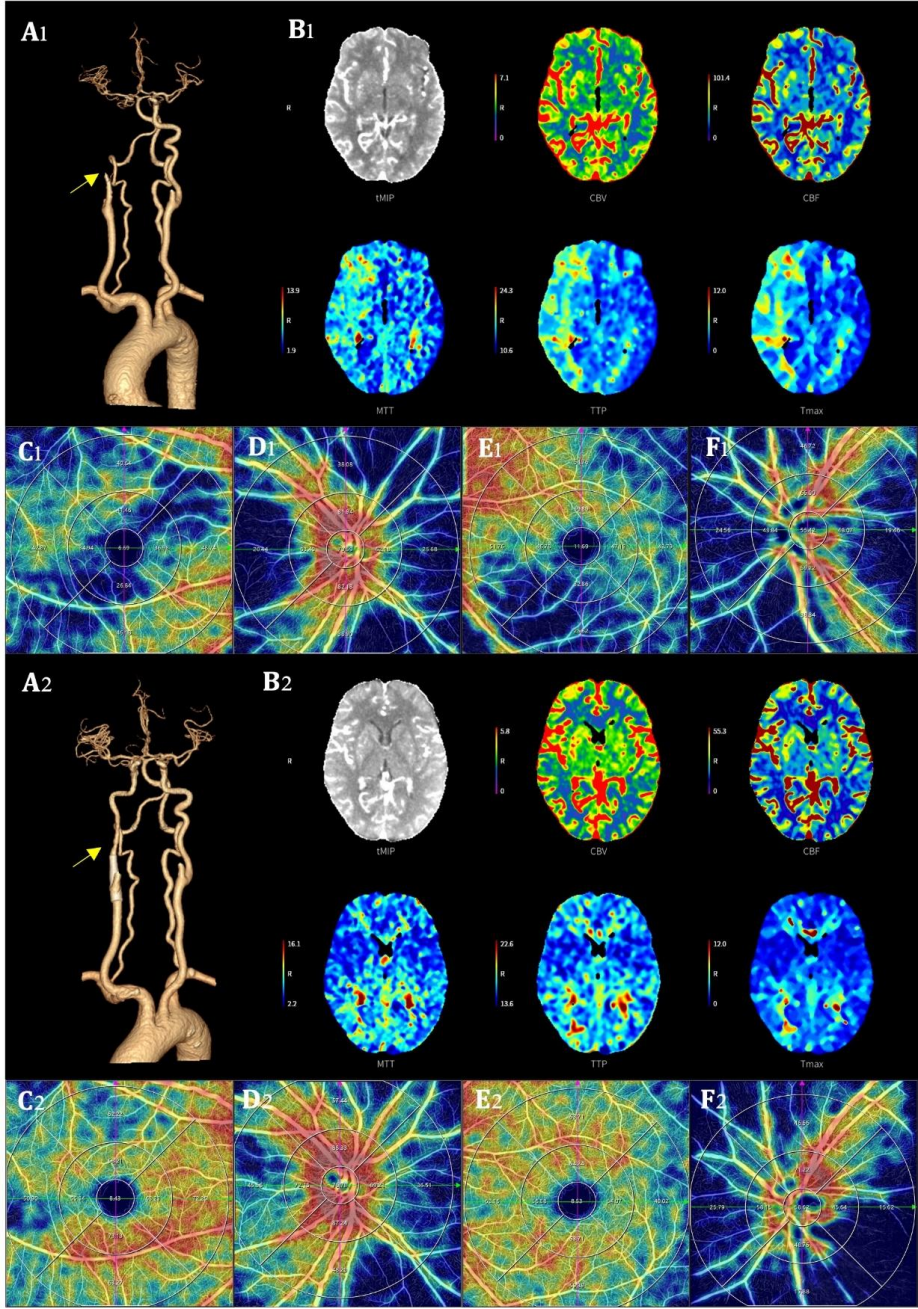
An example of OCTA findings in a CAS patient. A 66-year-old male accepted carotid artery stent surgery due to severe stenosis of the right carotid artery (>90%). Baseline (December 27^th^, 2023) CTA and CTP showed an occlusion of the right ICA (C2-C7 segments) (yellow arrow, A1), and extensive hypoperfusion in the right hemisphere (B1). In his pre-operative OCTA images, the SRCP VD in the macular (C1, E1) and optic disc (D1, F1) regions of both eyes were significantly reduced, especially seen in the right eye (C1*vs*. D1, E1*vs*. F1). Three days after the surgery (January 12^th^, 2024), the follow-up CTA image revealed that the occluded right ICA was successfully recanalized (yellow arrow, A2 *vs*. A1), and the baseline intracranial hypoperfusion was reversed to normal (B2 *vs*. B1). The post-operative OCTA images showed significant improvement of the SRCP VD of the macular (C2 *vs.* C1, E2 *vs.* E1) and optic disc areas (D2 *vs.* D1, F2 *vs.* F1) in his both eyes, indicating that the blood flow perfusion of the retina in both eyes has been restored. CAS, carotid artery stenosis; CTA, computer tomography angiography; CTP, computer tomography perfusion; ICA, internal carotid artery; OCTA, optical coherence tomography angiography; SRCP, superficial retinal capillary plexus; VD, vessel density.
